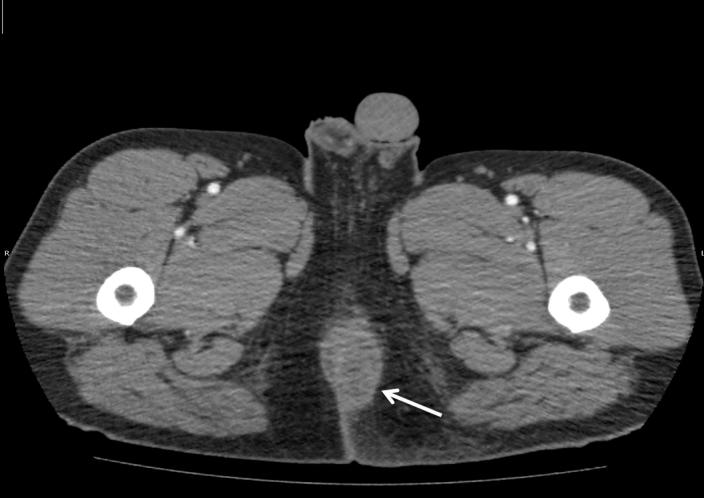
Pelvic CT showing peri-rectal fluid collection (white arrow).
